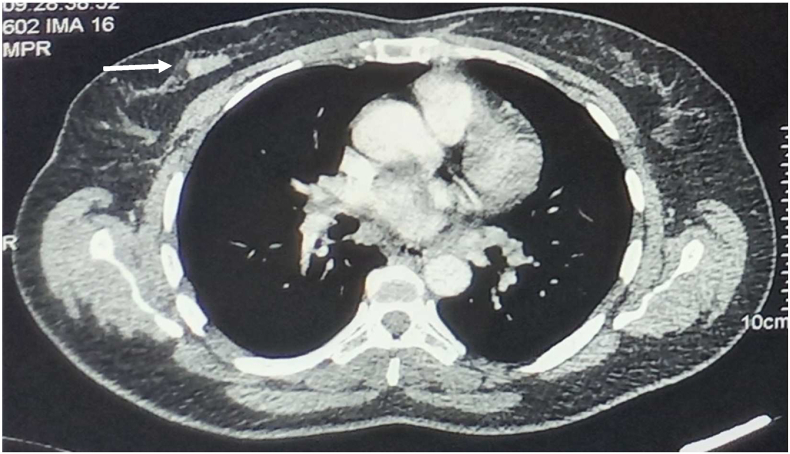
A thoracic CT scan showing a right breast lump (white arrow).
